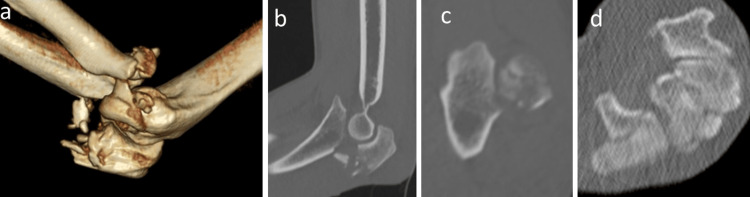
Preoperative CT. (a) Three-dimensional image of the left elbow. This figure shows fracture of the olecranon and radial head and dislocation of the humeroradial joint. (b) Sagittal plane of the left elbow. This figure shows intra-articular fractures of olecranon. (c) Axial plane of the left proximal radioulnar joint. This figure shows proximal radioulnar joint which is not dislocated. (d) Axial plane of the left carpal bone. This figure shows fracture of the hook of the hamate.
